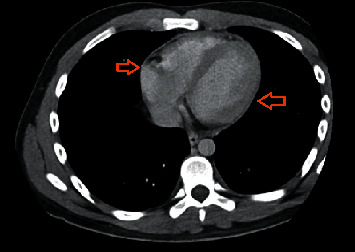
Computed tomography (CT) of the chest demonstrating pericardial thickening.
